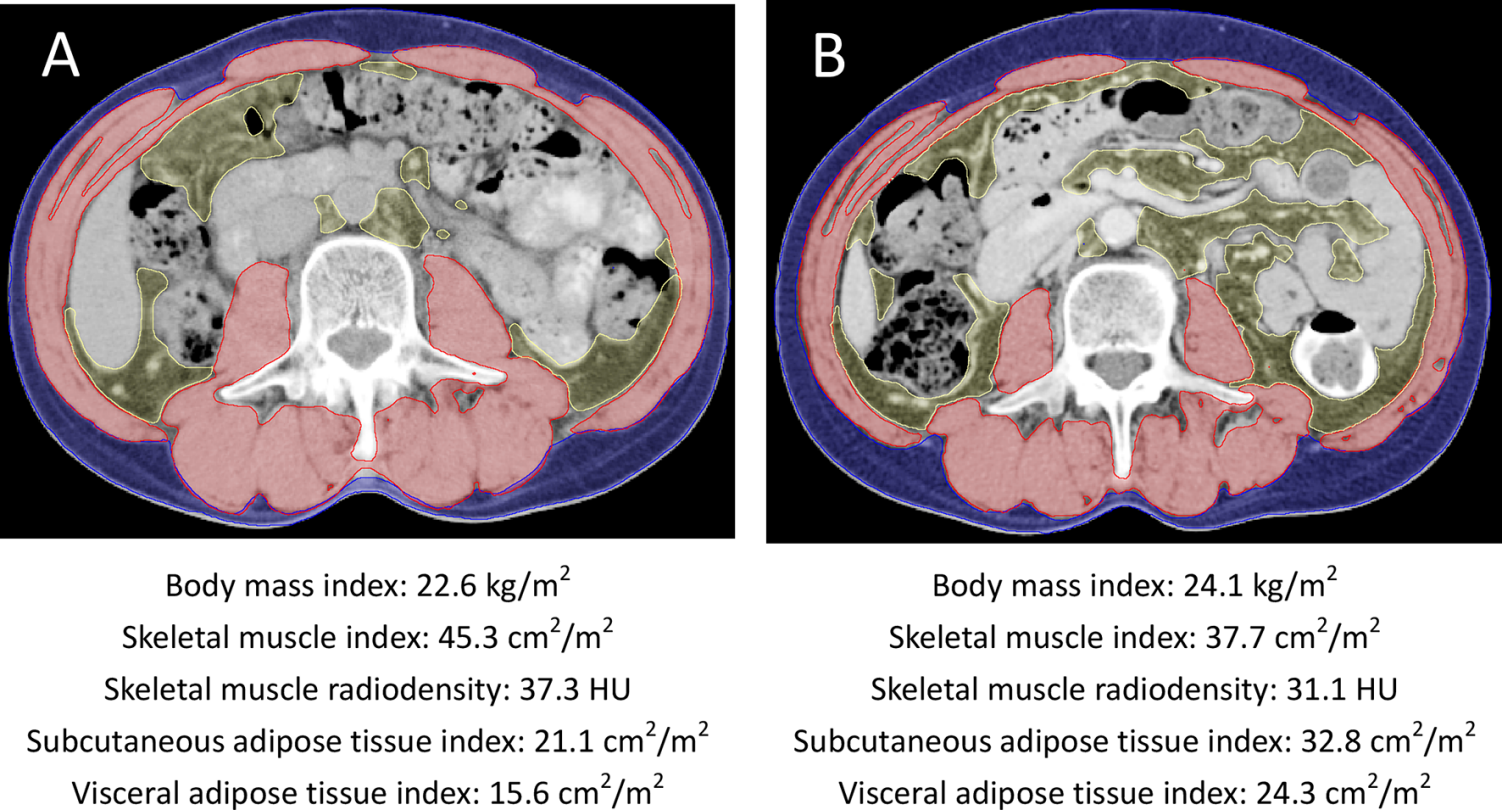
Axial cross-sectional CT images at the L3 vertebral level (A) at baseline and (B) at 6 months after initiation of radiotherapy and androgen deprivation therapy from one patient. The skeletal muscle areas, subcutaneous adipose tissue areas, and visceral adipose tissue areas were outlined in red, blue, and yellow, respectively. This patient had increased body mass index, subcutaneous and visceral adipose tissue indexes and decreased skeletal muscle index during radiotherapy and androgen deprivation therapy.
